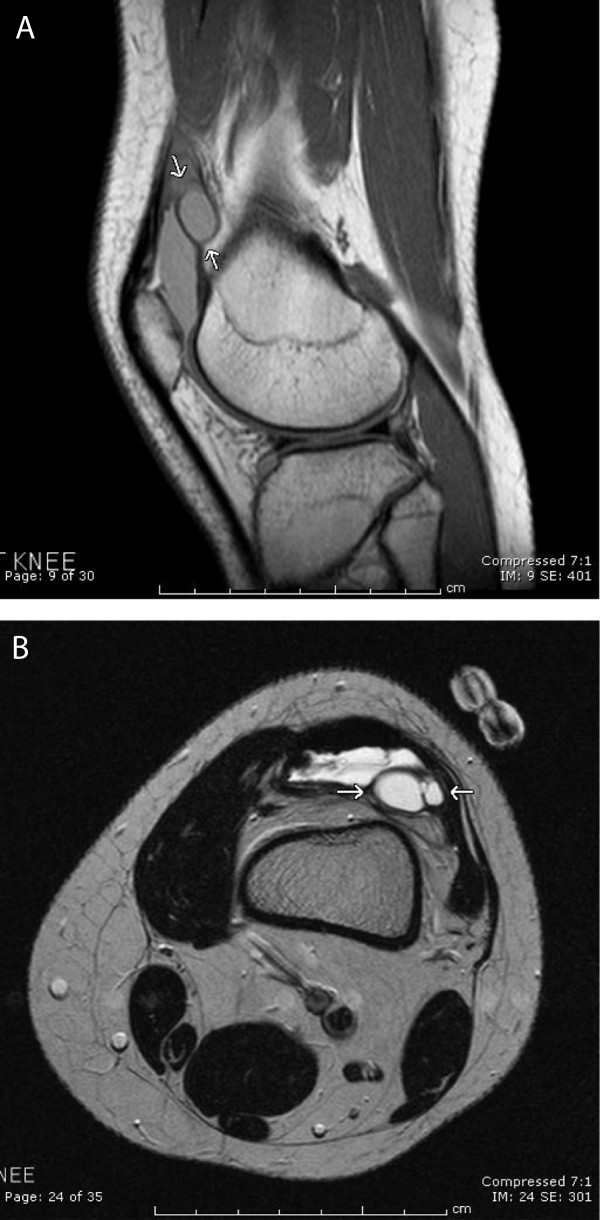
MRI of intra-articular ganglion cyst. (A) Axial T2-weighted image shows a mildly septated T2 hyperintense fluid intensity lesion within or projecting into the superolateral aspect of the knee. (B) Sagittal proton density weighted image shows a slightly elongated fluid intensity area adjacent to or within the upper aspect of the joint space.
